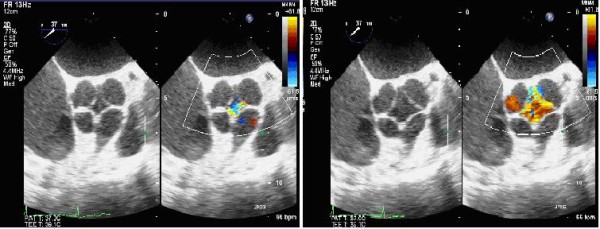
Transesophageal echocardiogram (TEE) short axis views in the vicinity of the aortic valve plane showed a quadricuspid aortic valve. The four cusps demonstrated a mild degree of calcification but a well-preserved motion. There was a central jet on the color Doppler examination, which is consistent with the severe aortic insufficiency (AI).
